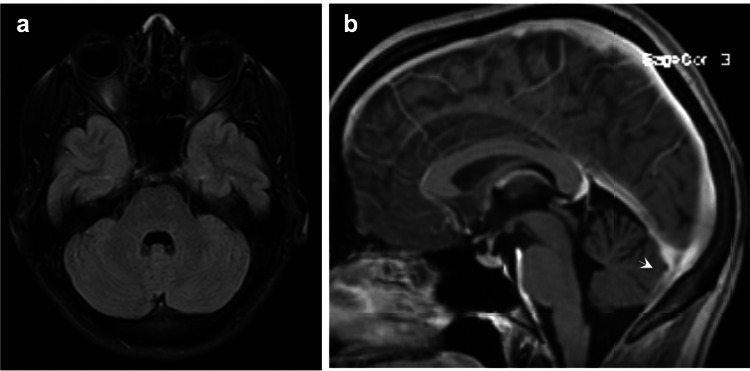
One year after the initial cerebral MRI, (a) T2WI shows complete resolution of the signal alteration in the vermis (arrow), medial cerebellar hemispheres, and occipito-polar region (edema). (b) Contrast-enhanced T1WI reveals reduced thickening and pachymeningeal enhancement previously observed in the region of the torcula (arrow). T2WI: T2-weighted imaging; T1WI: T1-weighted imaging.
